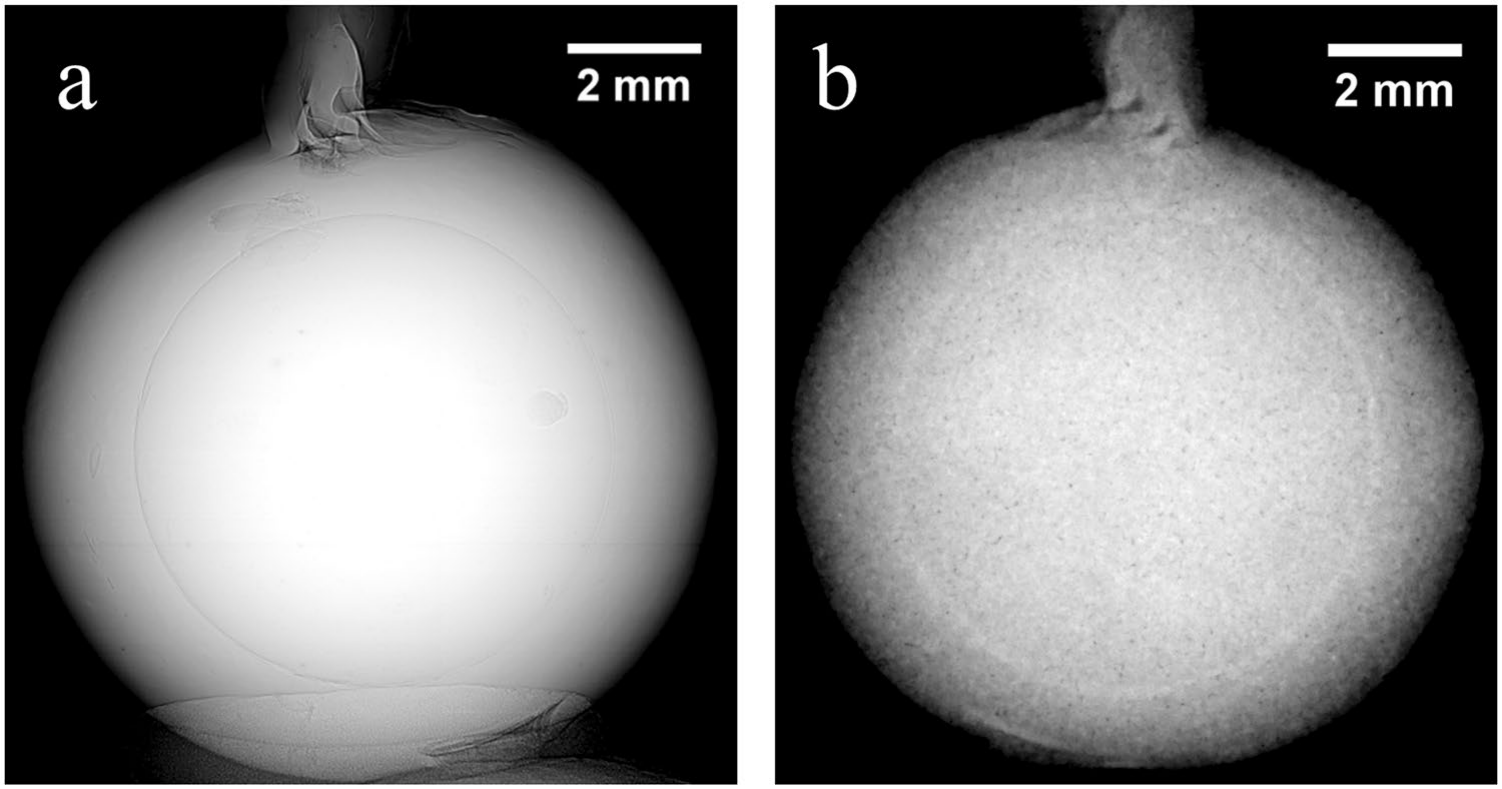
X-ray PC (a) and neutron (b) radiographs of the beaded saltwater pearl CP −17. A ring, due to contact surface between nacre and bead, is recognized in both radiographs.
